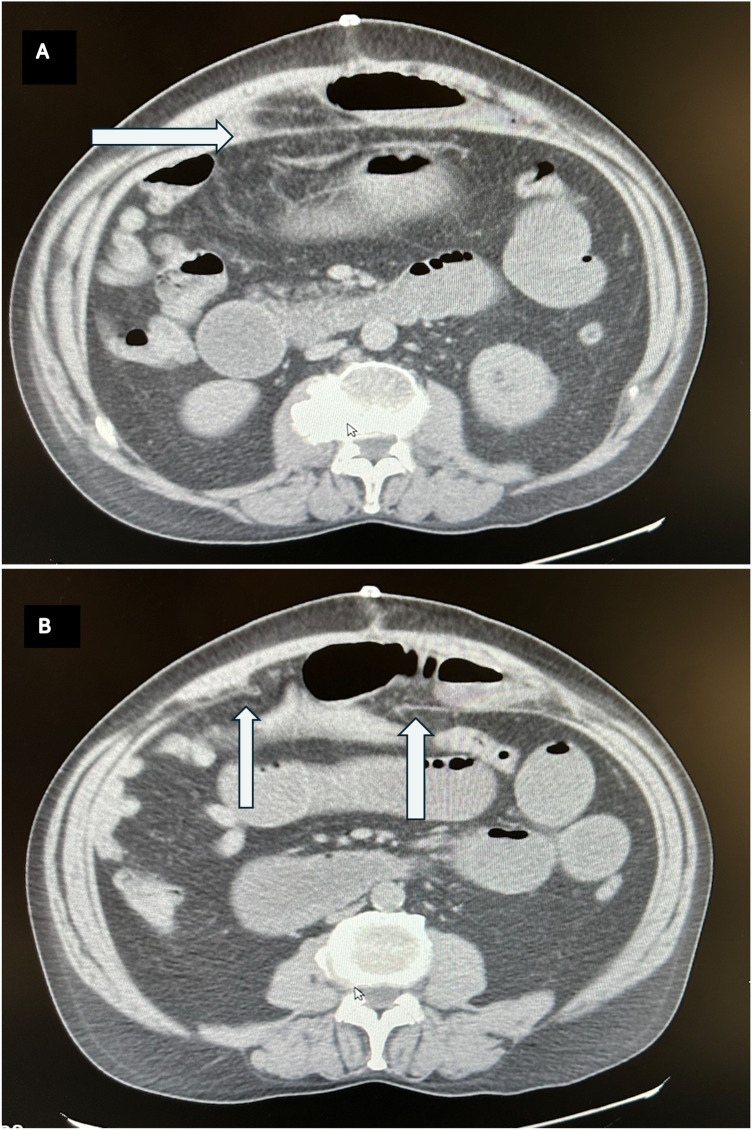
(A,B) Abdominal CT scan images showing the interparietal hernia. White arrows: The posterior rectus sheath. (A) Intact, (B) free edges surrounding the orifice.
